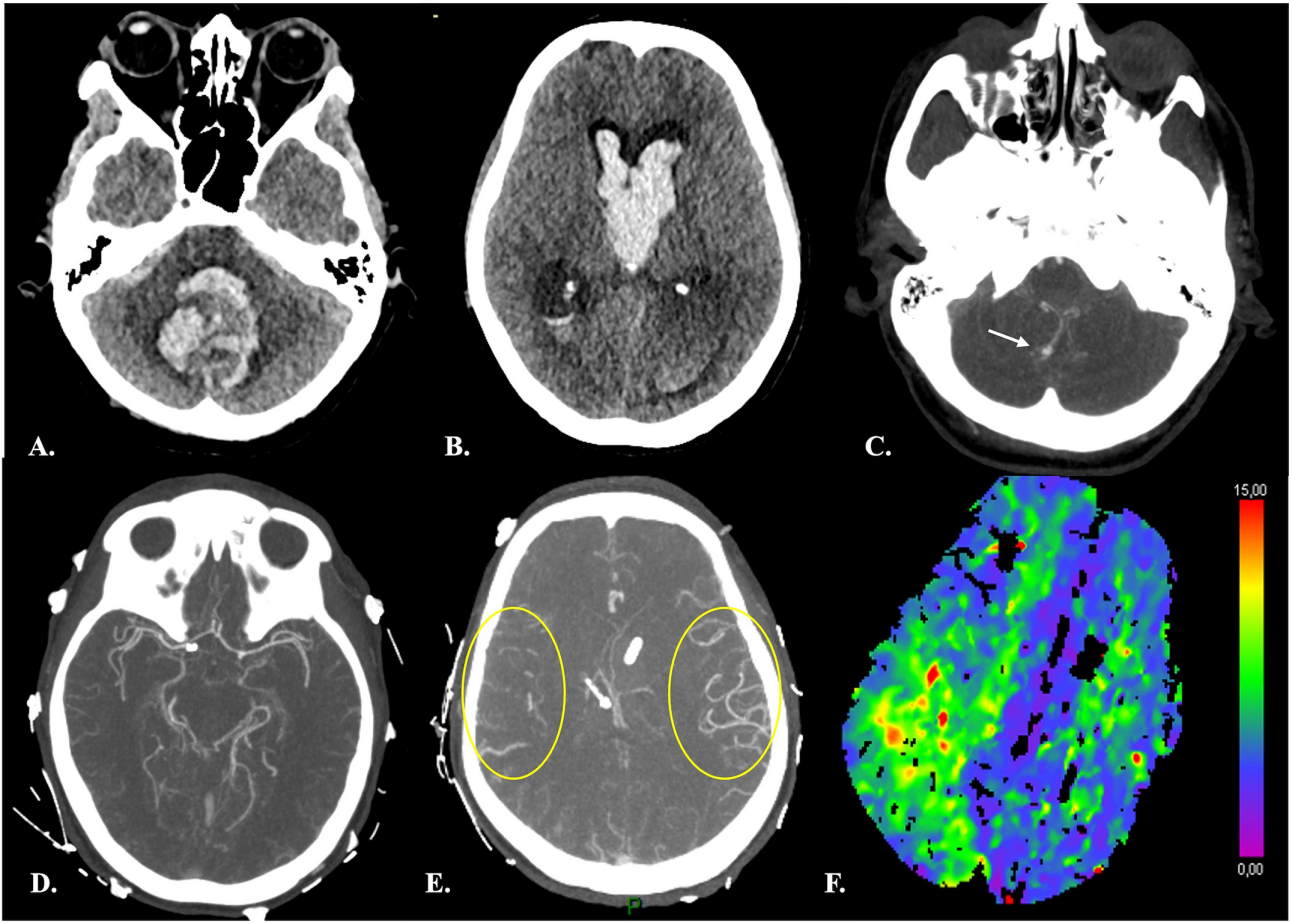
Distal vasospasm and corresponding hypoperfusion in a 42-yr-old female with Fisher 4 aSAH. (A–B) Brain NECT at admission showed a large hematoma centered on the fourth ventricle, intraventricular hemorrhage and hydrocephalus. CTA (C) demonstrated a ruptured right PICA aneurysm (*arrow*) and an unruptured right carotid-ophtalmic aneurysm (not shown). (D–E) At day 7 after aSAH and endovascular treatment of both aneurysms, CTA disclosed vasospasm of distal right MCA branches (*yellow circles in E, for comparison with contralateral MCA territory*). (F) CTP revealed prolonged TTD consistent with hypoperfusion in the corresponding ipsilateral sylvian territory.
